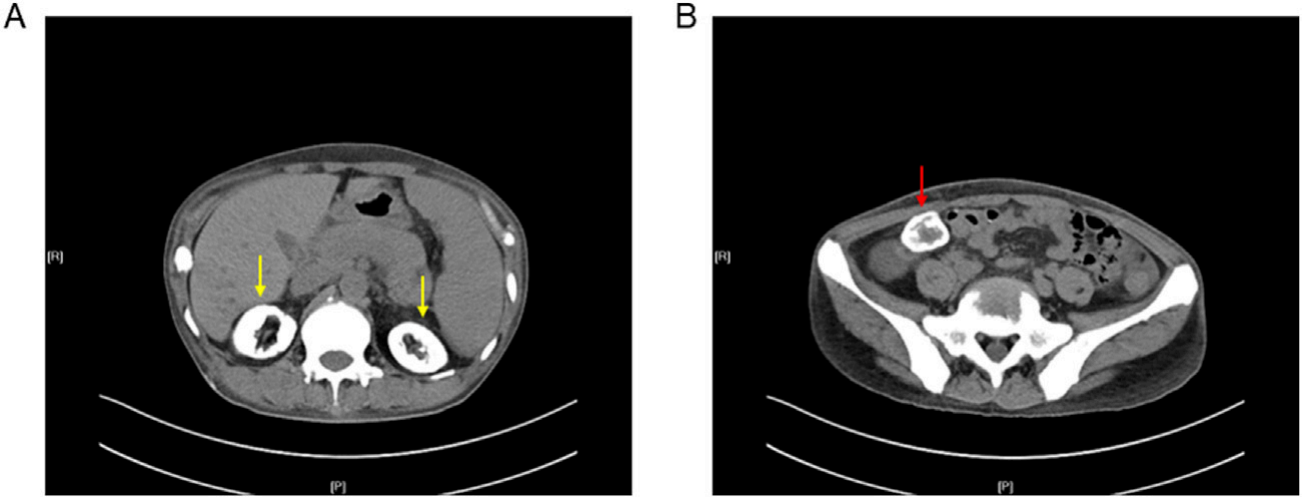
Computed tomography of the abdomen and pelvis showed nephrocalcinosis in (A) both kidneys (yellow arrow) and (B) transplanted kidney (red arrow).
